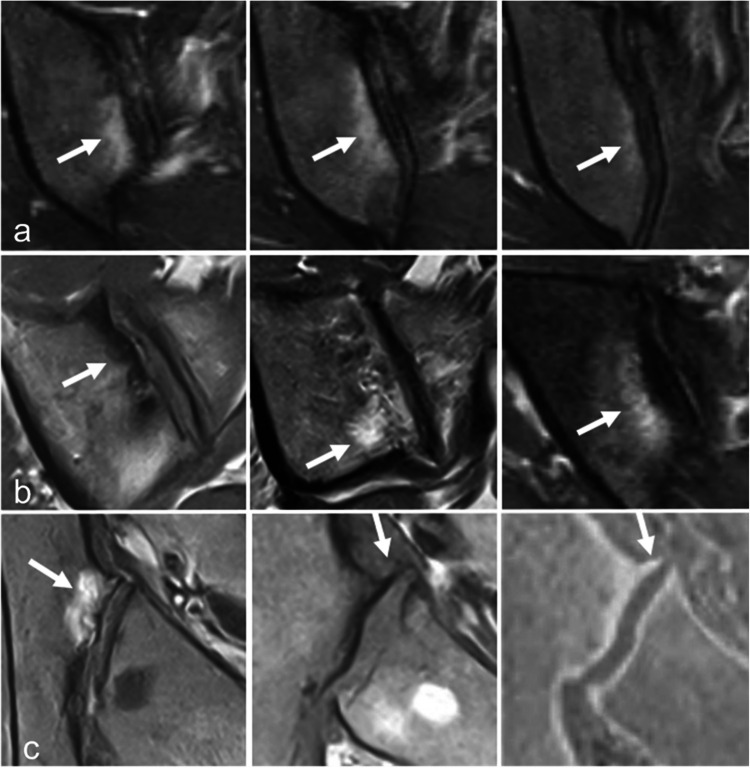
Mechanical changes at the anterior aspect of the middle third of a right SIJ. a Edematous changes in the iliac bone (arrow) on three contiguous coronal oblique T2-weighted images in a 41-year-old woman. b Sclerotic, fatty, and mixed subchondral changes (arrows) on coronal oblique T1-, T1-, and fat-saturated (FS) T2-weighted images in three different patients, respectively. c Subchondral bone marrow edema (arrow) on an axial oblique FS T2-weighted image and osteophytes (arrow) on T1- and StarVIBE T1-weighted images in the same patient as in a
